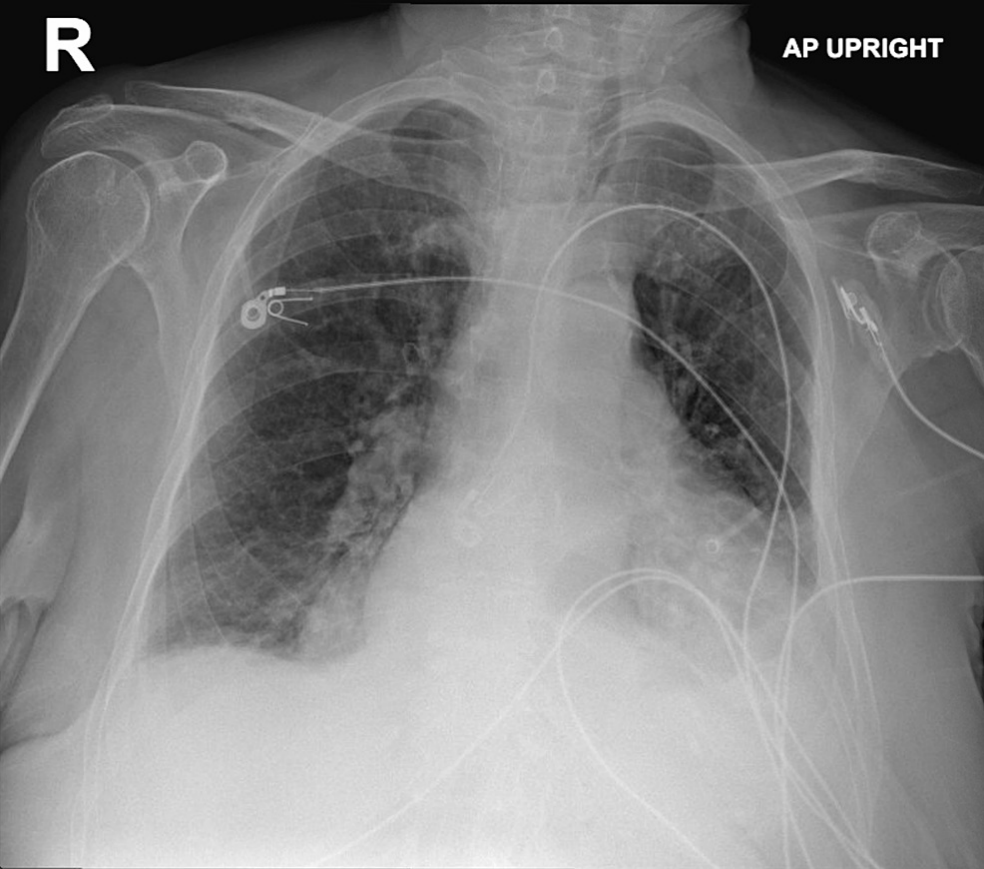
Initial chest X-ray showing cardiomegaly and pleural effusion
